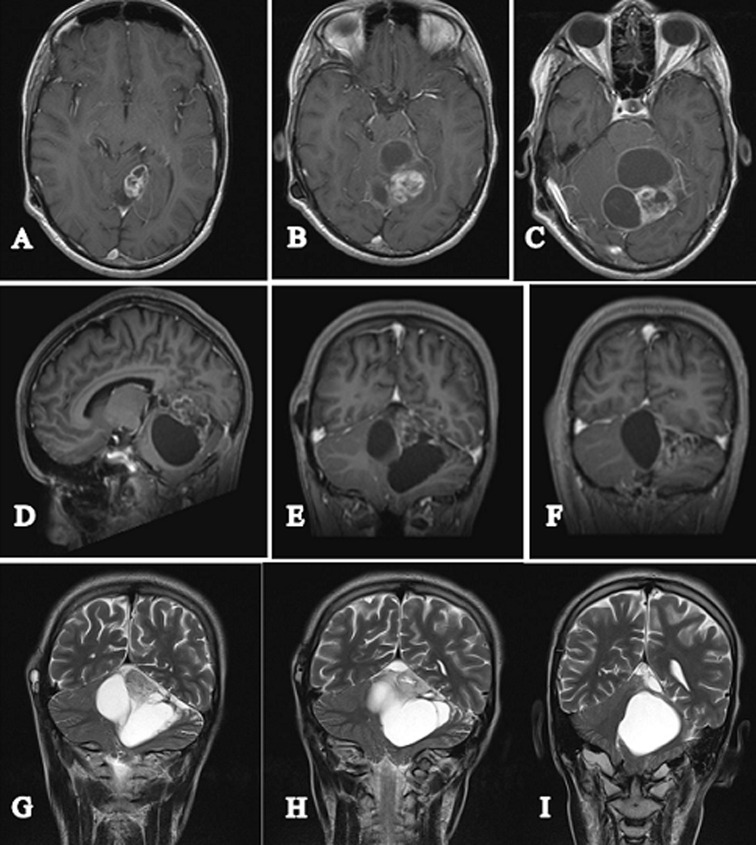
brain MRI control showing a decrease in the size of the tumor process after radiation therapy; axial section T1 injected (A+B+C) sagittal slice (D) and coronal sliceS (E+F), coronal sections T2 (G+H+I)
